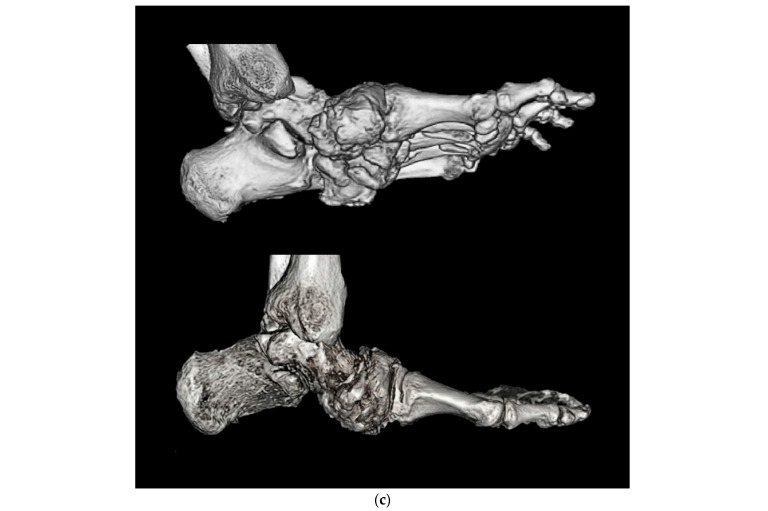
Comprehensive assessment of Charcot Neuro-osteoarthropathy (CNO). (a) Clinical photograph showing the classic “rocker-bottom” deformity with a plantar midfoot ulcer, resulting from uncorrected structural collapse. (b) Pedobarographic assessment revealing a pathological shift in peak plantar pressure to the midfoot (red zone), confirming the functional impact of the deformity. (c) Weight-bearing computed tomography (WBCT) 3D rendering of the same foot. This modality elucidates the underlying osseous destruction and joint subluxation under load that drives the biomechanical failure seen in (b) and the clinical outcome seen in (a).
